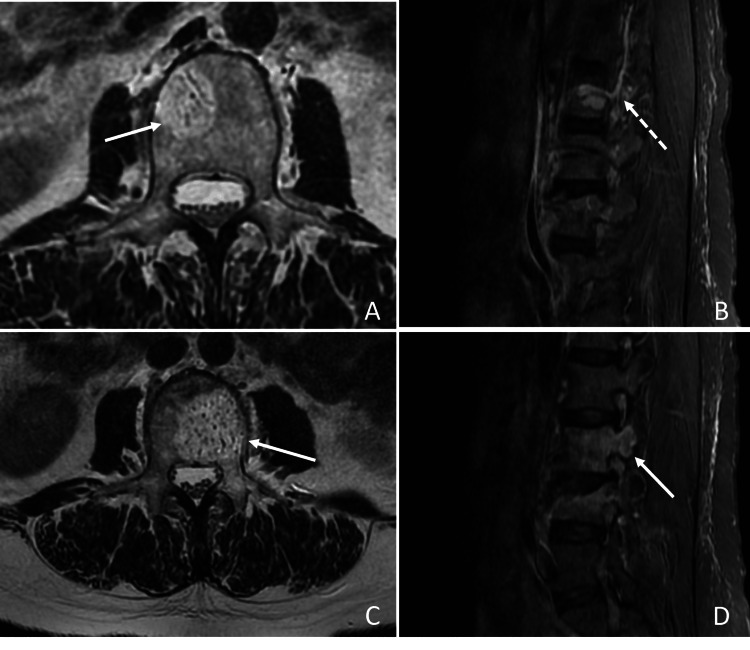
Magnetic resonance imaging (MRI) of the lumbar spine A and C, T2-weighted axial sequences; B and D, STIR (Short Tau Inversion Recovery) sagittal sequences. The MRI confirmed the presence of two adjacent atypical haemangiomatous neoformations at L2 and L3 without invasion or compression of the spinal canal. A. T2-2 axial sequence of L2. The fat-suppressed sequence demonstrated an important fat component within the neoformation (white arrow). B. STIR sagittal sequence of the lumbar spine, paramedian right. The right L2 pedicle involvement was found (hatched arrow). C. T2-w axial sequence of L3. Extending and invading the left peduncle (white arrow) was a critical fat component. D. STIR sagittal sequence of the lumbar spine, paramedian left. The left L3 peduncle was hyperintensified due to the extension of the haemangioma (white arrow). The preoperative MRI showed no ballooning, cortex lysis, or extraosseous soft tissue extension.
